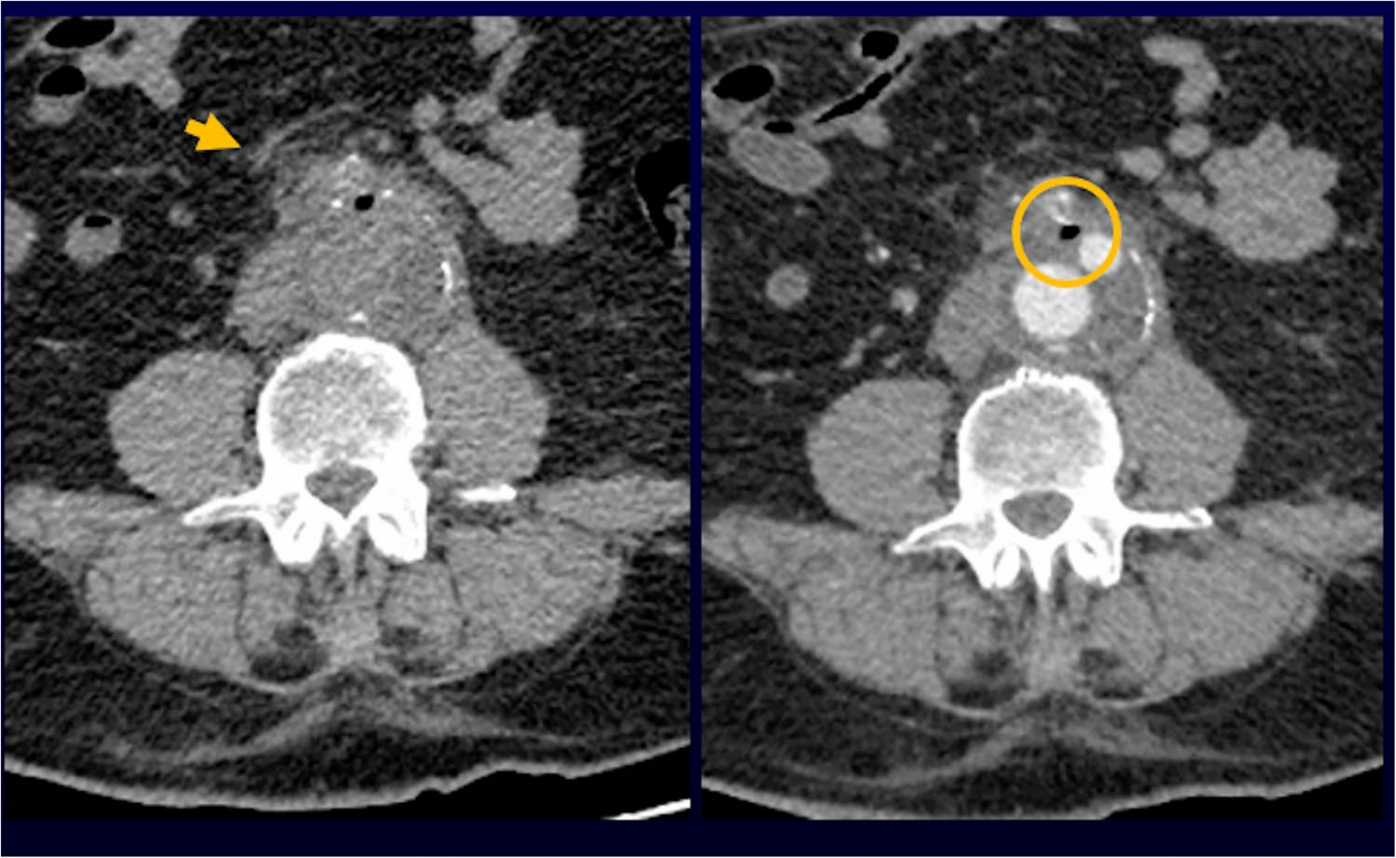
63-yo man who underwent open surgical repair of abdominal aortic aneurysm. CT images (a: unenhanced image and b: arterial-phase image) obtained 4 months after surgical treatment show aortic graft patency with peri-graft soft tissue stranding with peri-graft gas
